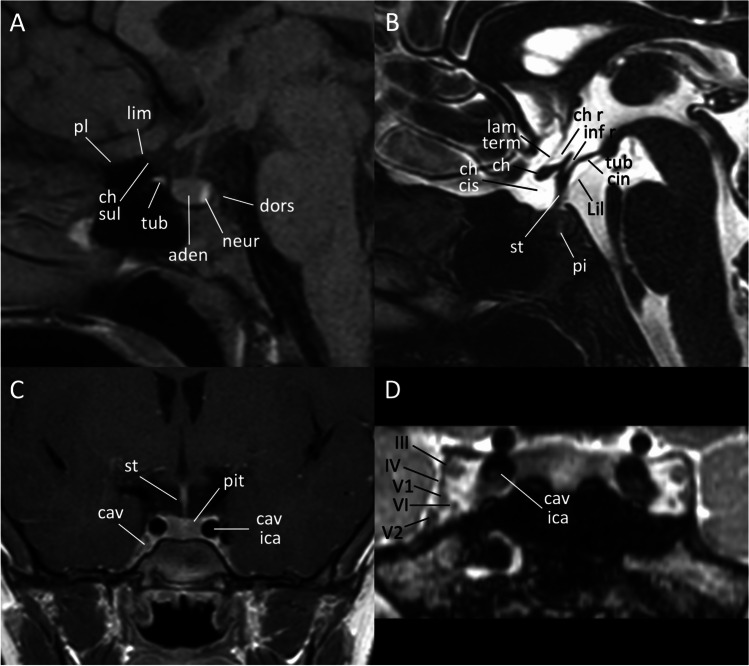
MR anatomy of the sellar region. Sagittal T1- (A), 3D high resolution T2- (sagittal reformat, B) and contrast-enhanced coronal T1-weighted (C) images; coronal reformat of a contrast-enhanced 3D balanced gradient echo sequence (D). *aden* adenohypophysis, *cav* cavernous sinus, *cav ica* cavernous segment of the internal carotid artery, *ch* optic chiasm, *ch cis* chiasmatic cistern, *ch r* chiasmatic recess, *ch sul* chiasmatic sulcus, *dors* dorsum sellae, *inf r* infundibular recess, *lam term* lamina terminalis, *Lil* Liliequist membrane, *lim* limbus sphenoidale, *neur* neurohypophysis, *pi* pars intermedia, *pit* pituitary gland, *pl* planum sphenoidale, *st* pituitary stalk, *tub* tuberculum sellae, *tub cin* tuber cinereum, *III* third cranial nerve, *IV* fourth cranial nerve, *V1* ophthalmic branch of the fifth cranial nerve, *V2* maxillary branch of the fifth cranial nerve, *VI* sixth cranial nerve
